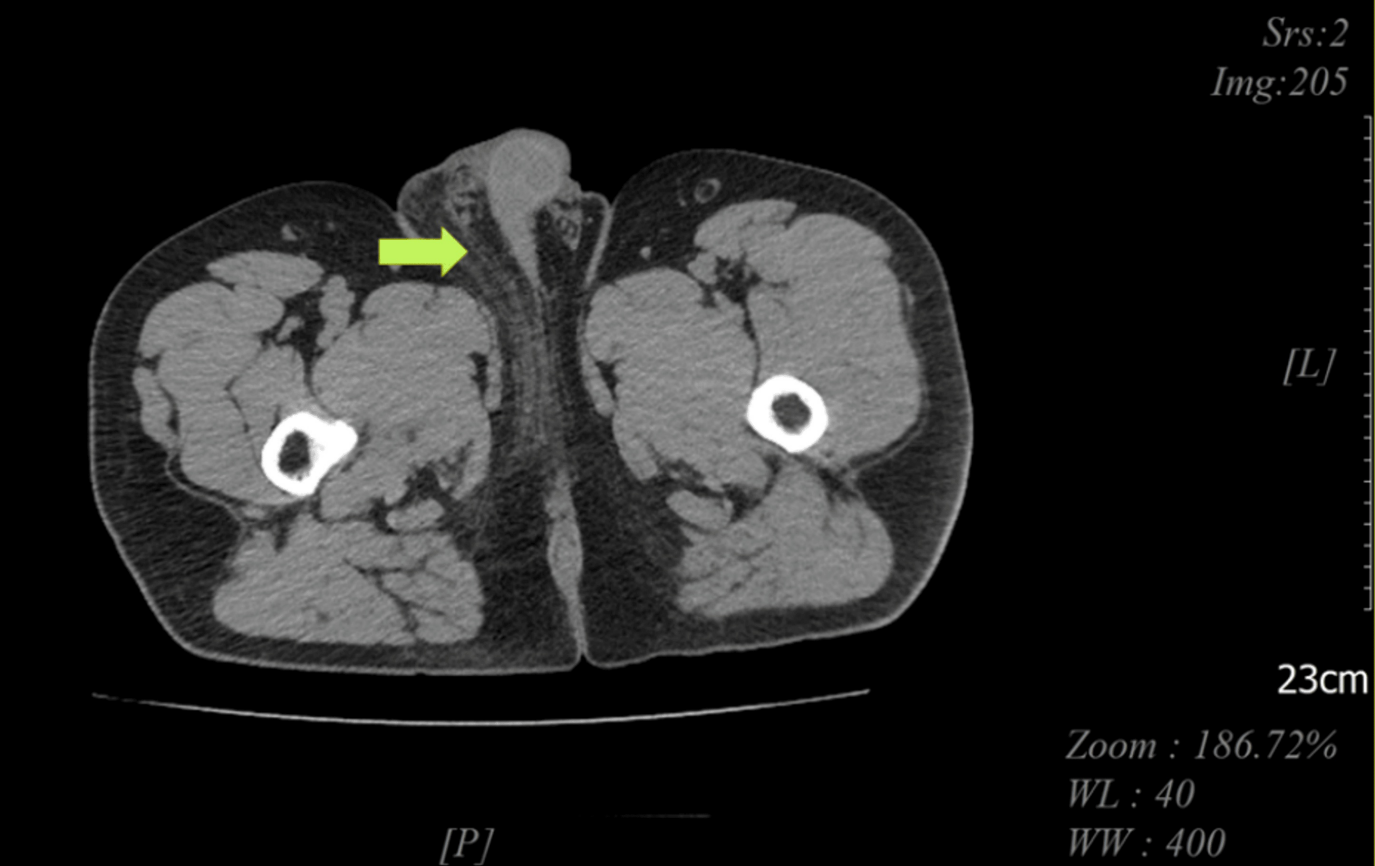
Abdominal/pelvic CT showing fat stranding in the perineum more evident on the right side than on the left (green arrow).
